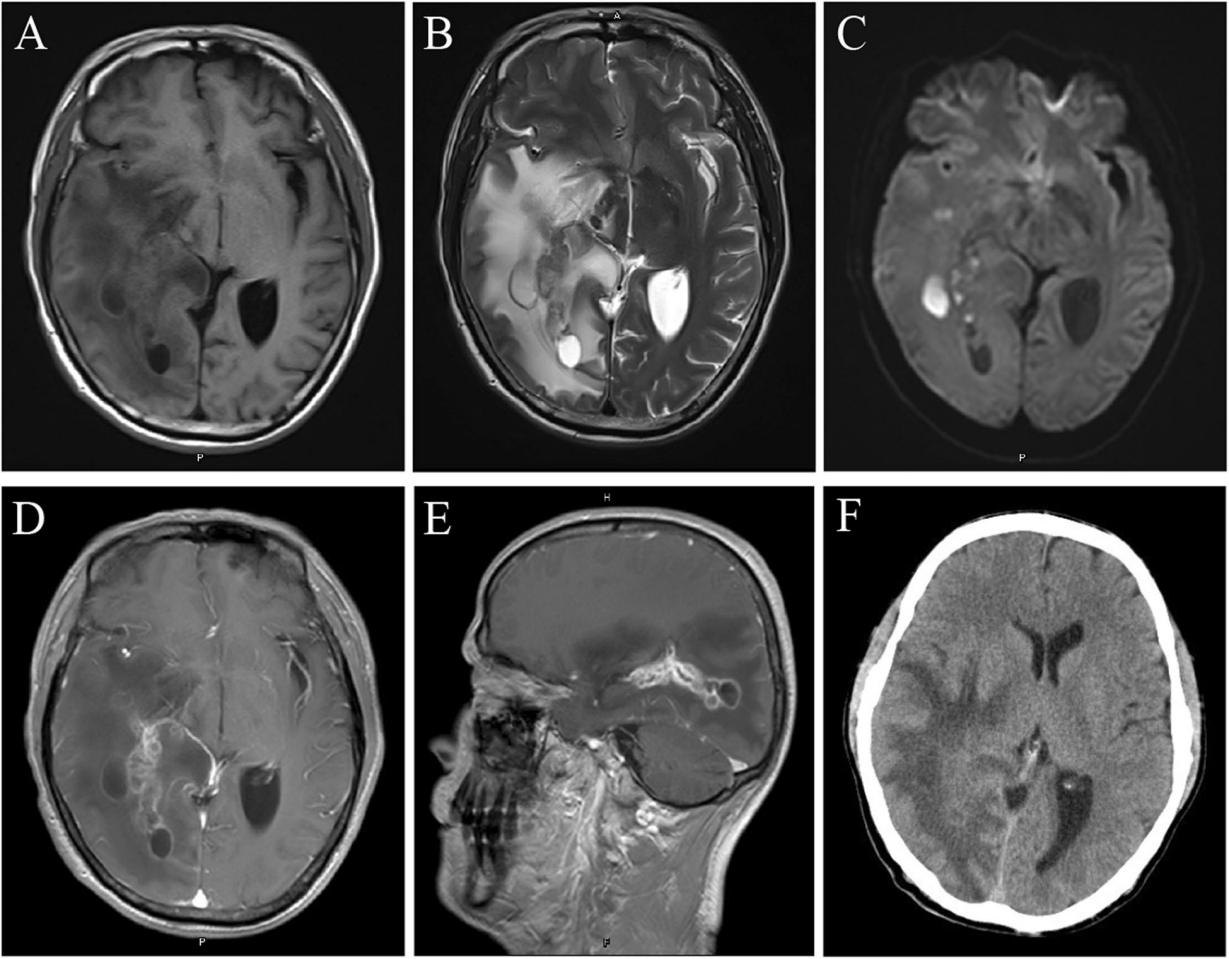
Head MRI and CT images before the second surgery. a-b Multiple irregular space-occupying lesions were found with slightly long T1-weighted and T2-weighted signal shadows in the right temporal lobe and occipital lobe that were surrounded by large patches of edema. c T2-weighted FLAIR showed high-intensity signals. d-e The signal intensity of the lesion ring wall of lesions was slightly increased after enhancement. f CT scan showed irregular low density edema shadows in the right temporal lobe and occipital lobe
